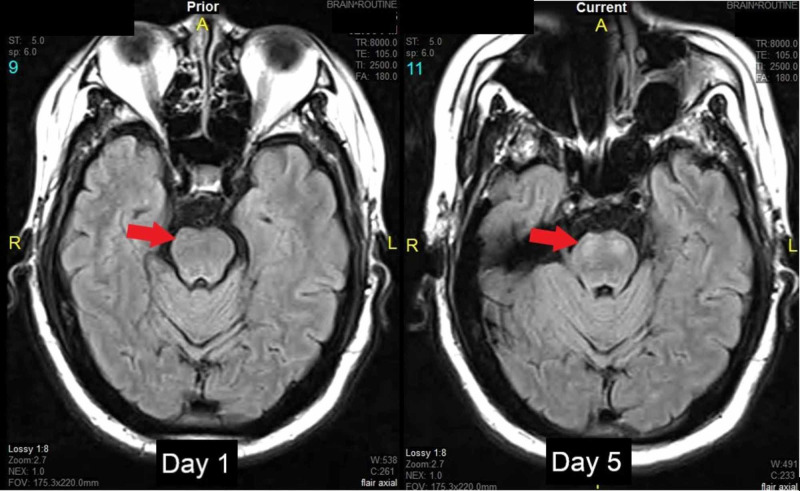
MRI slice of the pons on admission vs Day 5 Displayed are fluid-attenuated inversion recovery (FLAIR) sequences showing no acute pathology initially. Follow-up magnetic resonance imaging displayed bilateral basilar pontine infarcts not previously seen. These findings correlated with the patient’s clinical deterioration.
